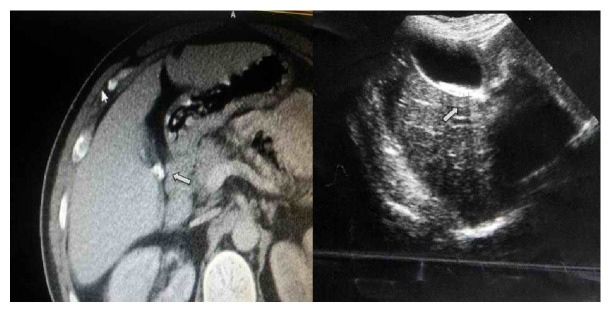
Parathyroid scintigraphy in patient with primary hyperparathyroidism: 99mTc sestamibi SPECT (white arrows).
